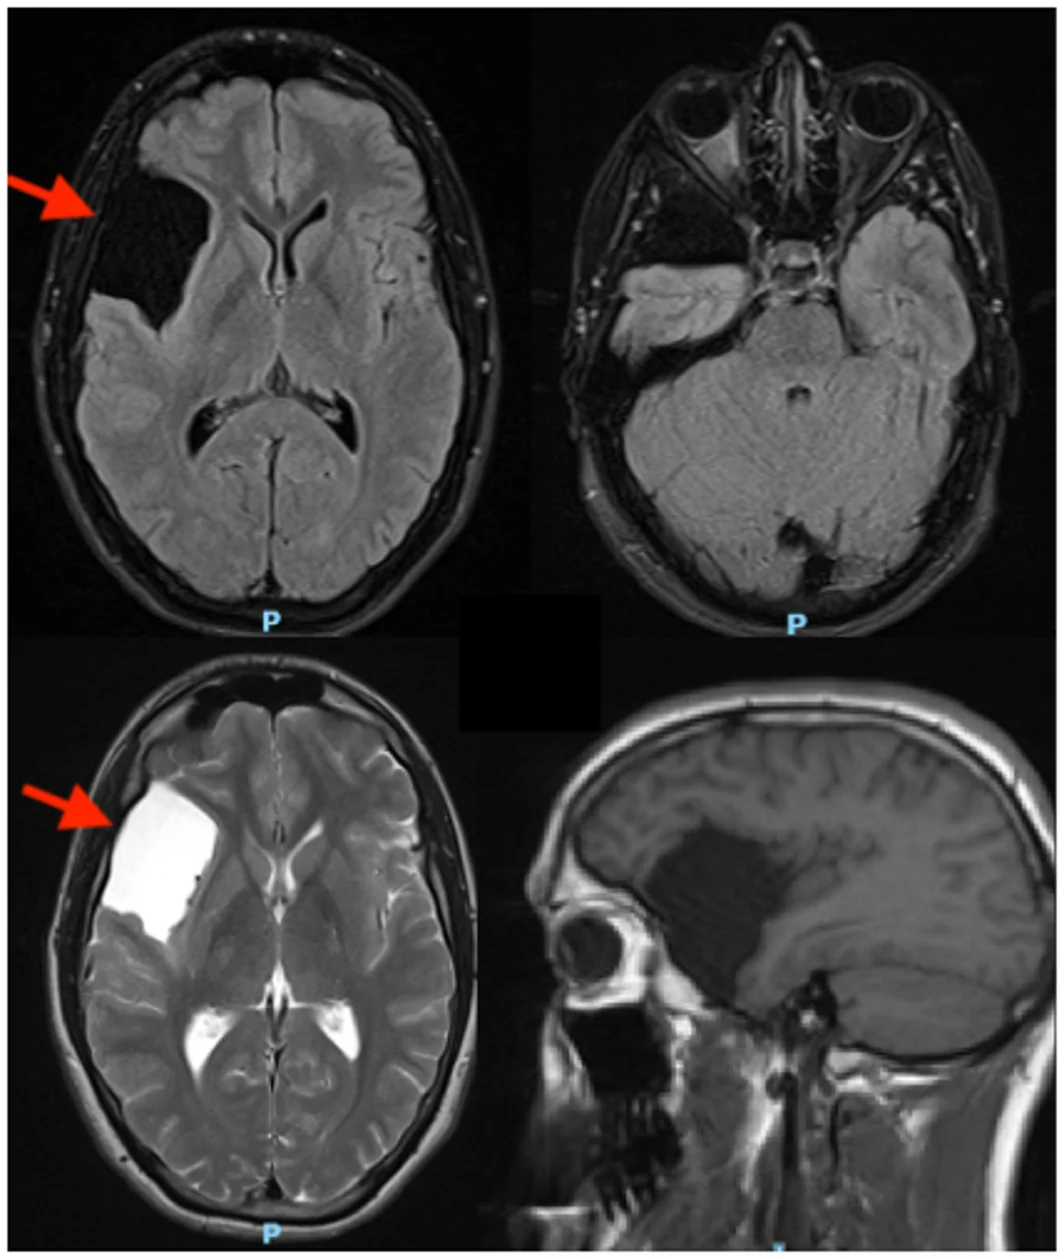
Magnetic Resonance Imaging demonstrating the arachnoid cyst located in the right temporal region.
